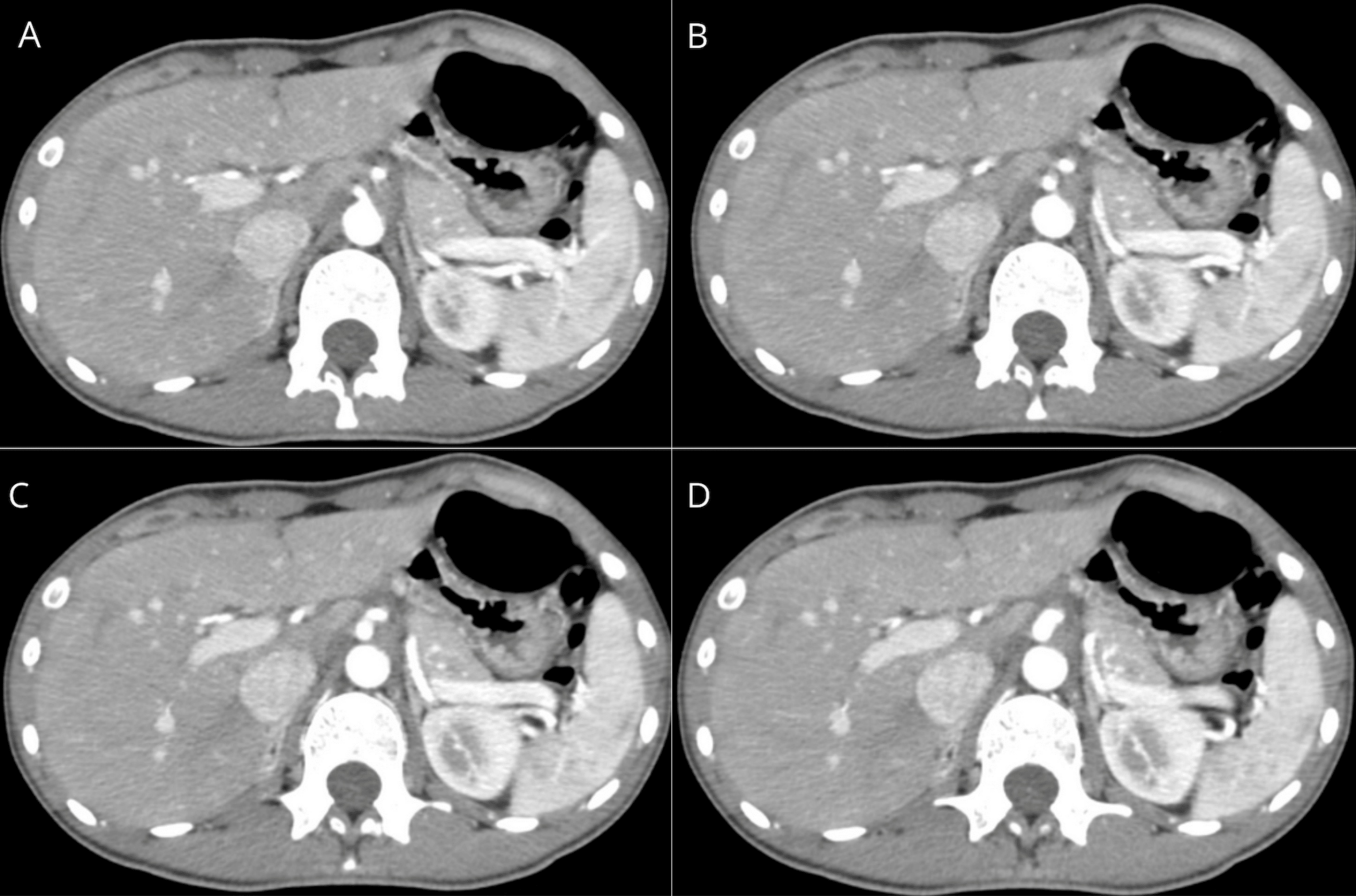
Sequential axial images from CT angiography showing significant luminal narrowing at the celiac trunk origin, with distal re-expansion and visible collateral circulation, suggestive of MALS. MALS: Median arcuate ligament syndrome
